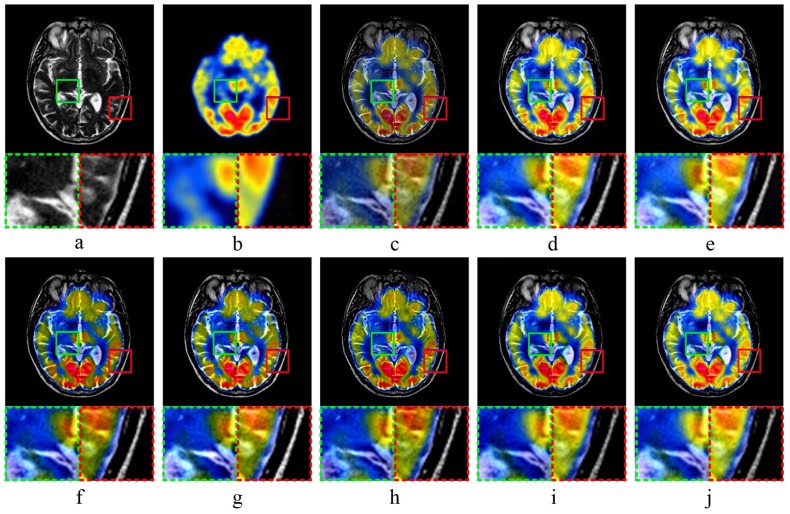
MR-PET image fusion Experiment 1: (a,b) source images; and (c–j) the fused image obtained by MST-SR, NSCT-PC, NSST-PCNN, ASR, CT, KIM, CNN-LIU, and the proposed method, respectively. Two partially enlarged images marked in green and red dashed frames correspond to the regions surrounded by green and red frames in the fused image.
